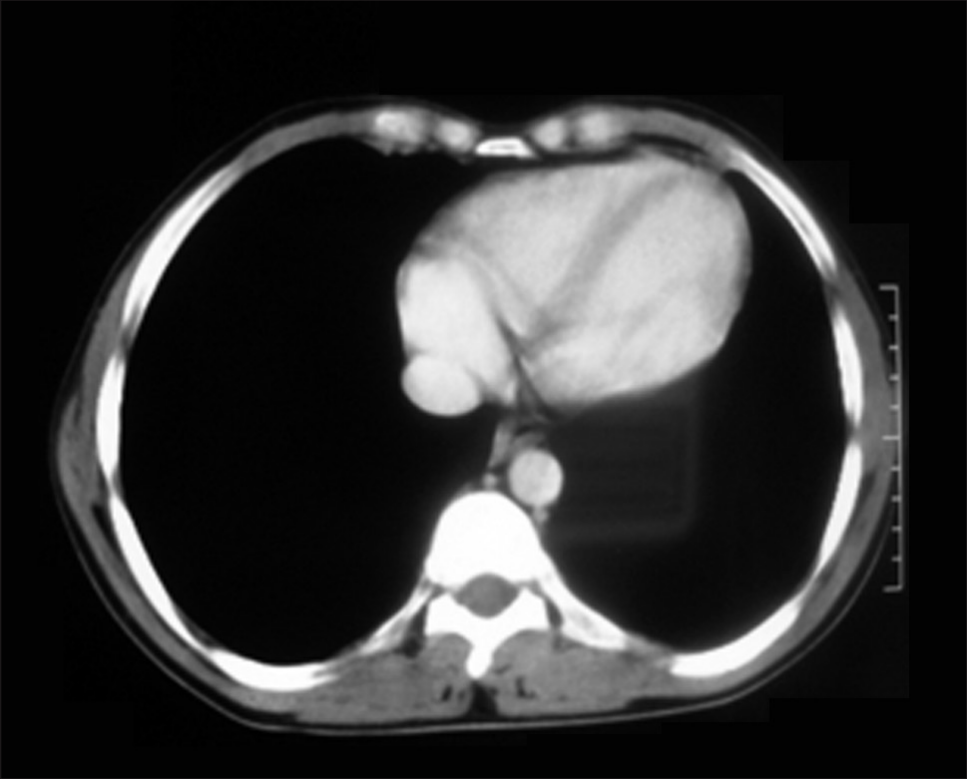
A repeat CT thorax shows the effusion has resolved following conservative management
